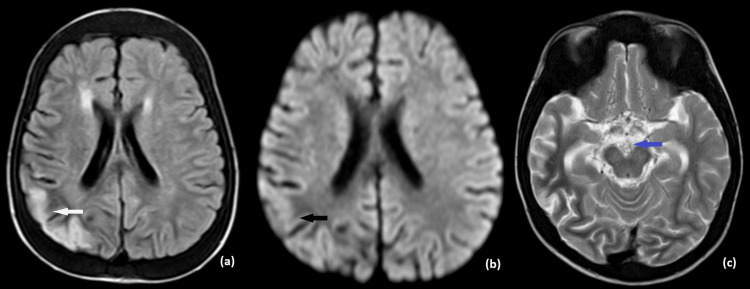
Axial sections of MRI brain. FLAIR (a) hyperintensity (white arrow) in the right parietal lobe with no restricted diffusion on DWI (b) (black arrow) suggestive of subacute infarct. Axial T2 weighted image (c) showing numerous vascular collateral channels in the basal cisterns (blue arrow).
